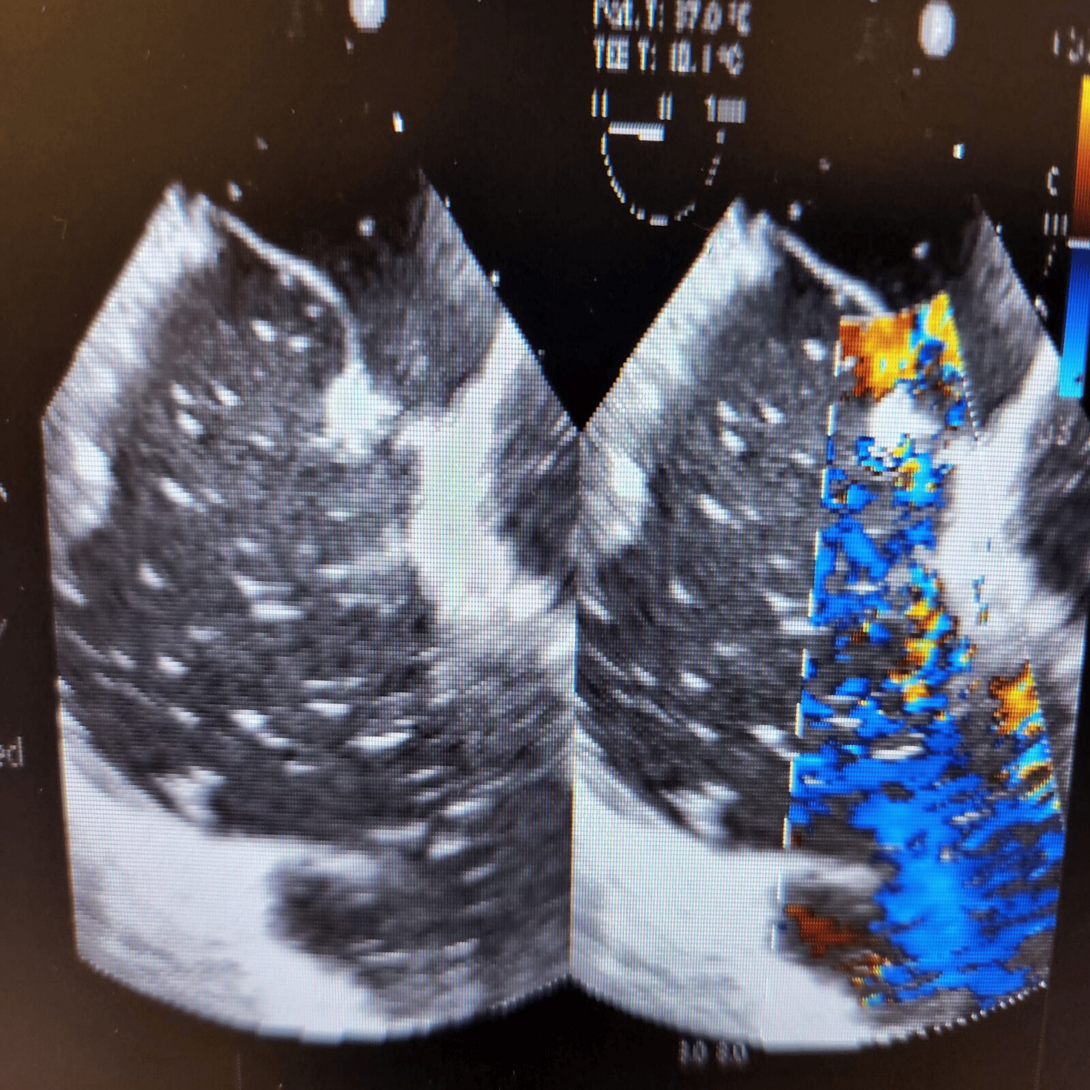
Gas bubbles seen passing through atrio-ventricular valves as seen in a 2D echo (echocardiogram)
